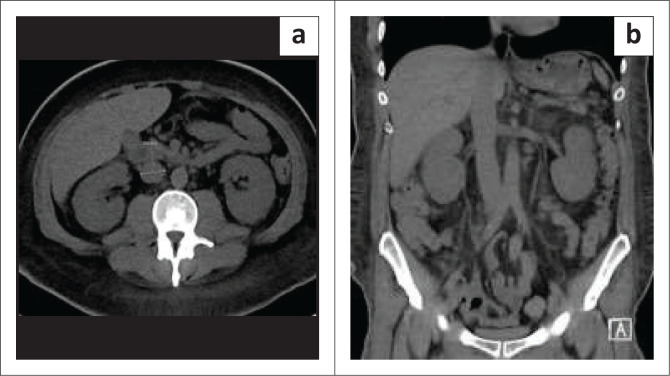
A 62-year-old with abdominal pain. Axial and coronal CT abdomen showing a prominent inferior vena cava measuring 29 mm in the infrarenal segment with anasarca probably from congestive heart failure.
